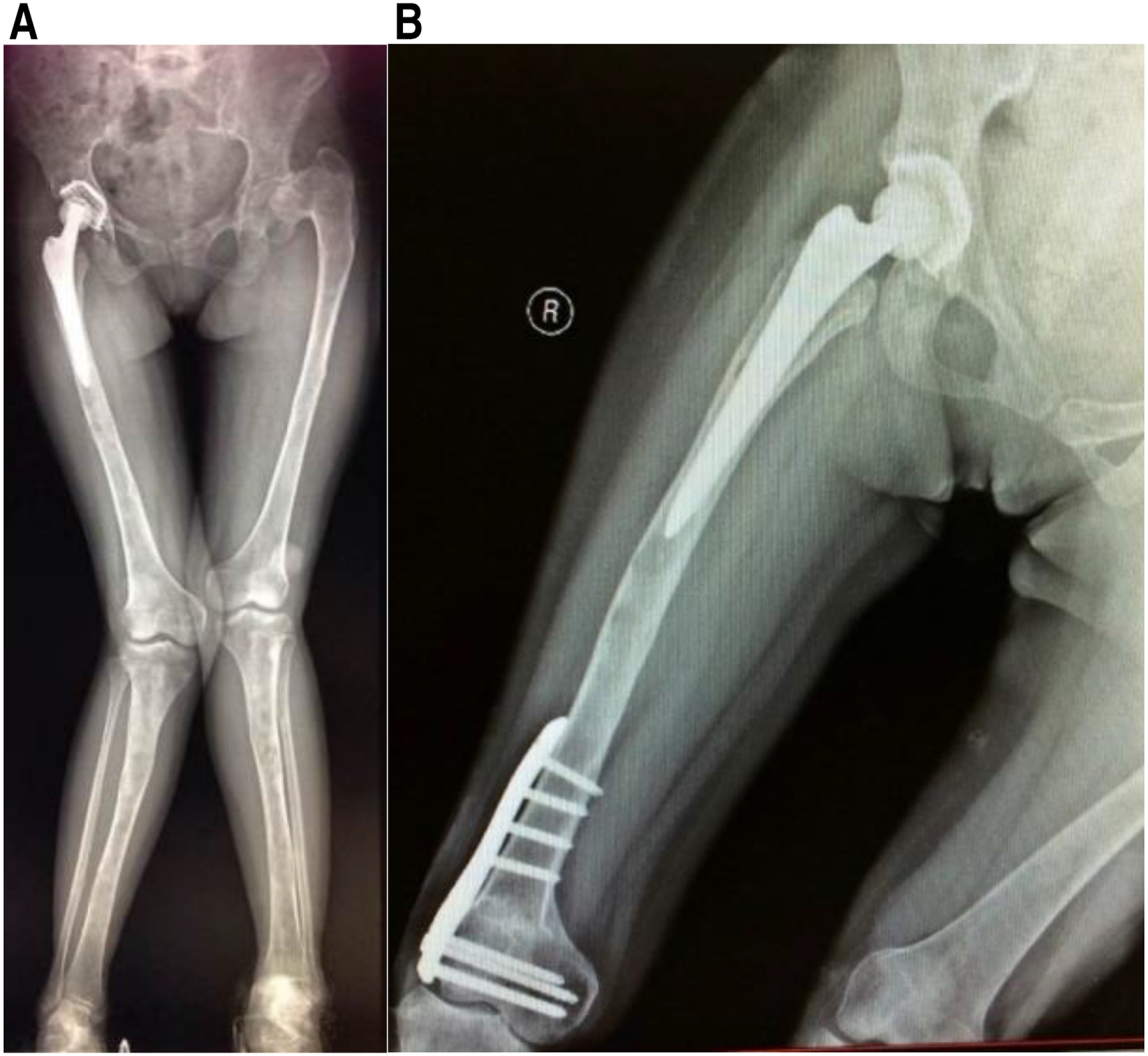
(A) Preoperative full-length lower limb radiograph. (B) X-ray after right knee valgus correction.
